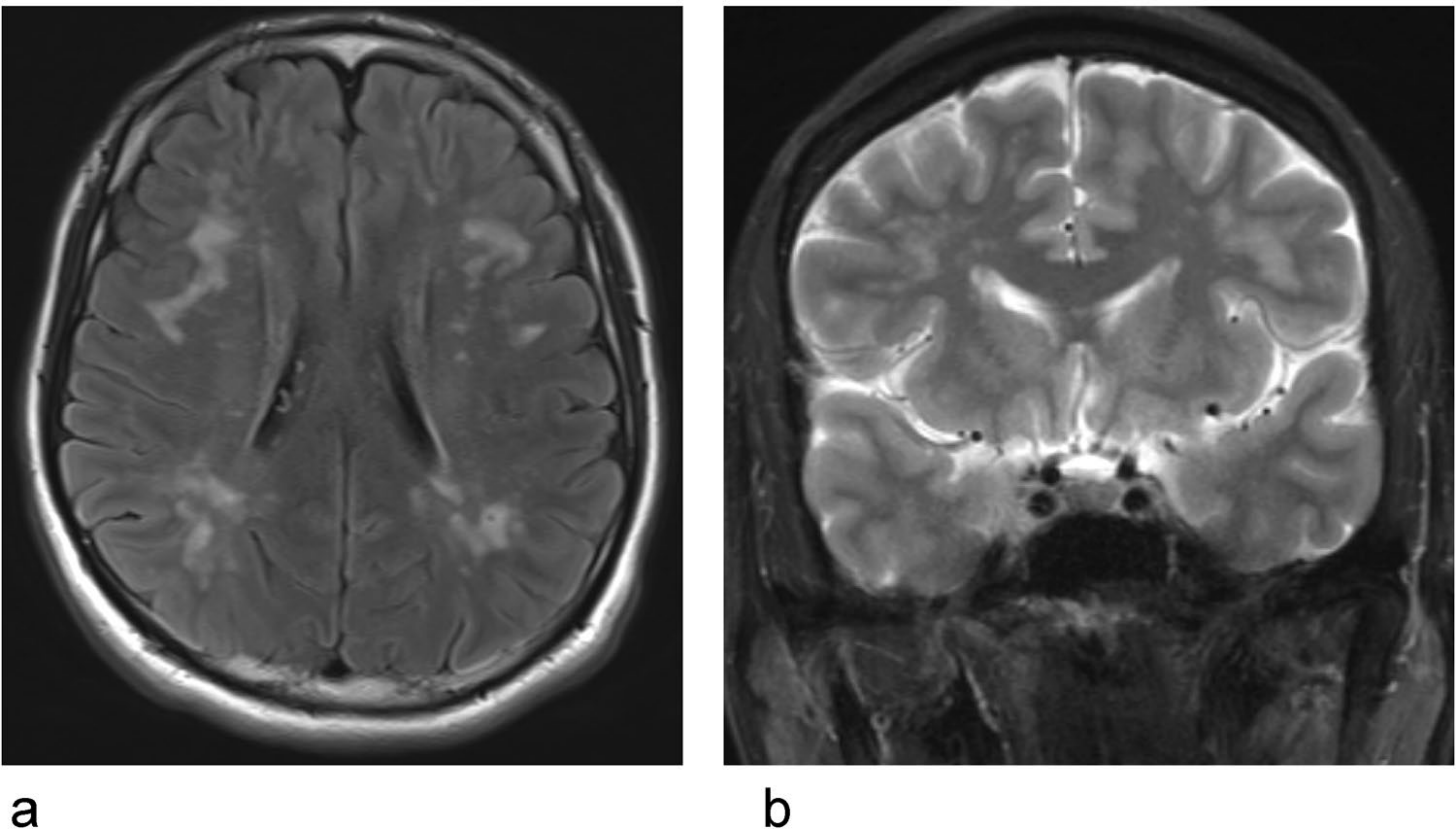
A case of small vessel disease diagnosed as CADASIL (cerebral autosomal dominant arteriopathy with subcortical infarcts and leukoencephalopathy) a FLAIR image, b T2-WI. A 5th decade male with severe migraine like symptom. Skin biopsy revealed GOM deposition and was diagnosed as CADASIL. His father had developed cerebral infarction in his 6th decade. Axial FLAIR image and coronal T2WI showing subcortical abnormal signal suggesting dilated perivascular space and surrounding gliosis
